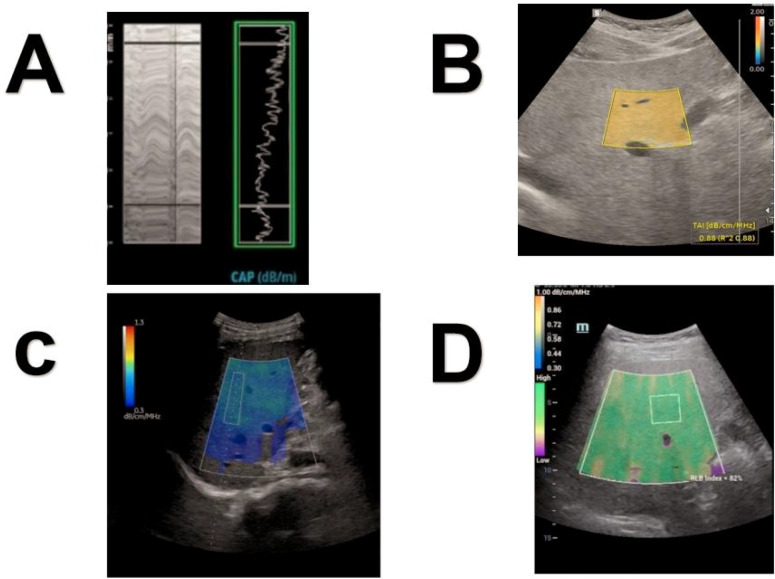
Representative ultrasound images of attenuation-based techniques for hepatic fat quantification. (A) CAP (Controlled Attenuation Parameter); (B) TAI (Tissue Attenuation Imaging); (C) UGAP (Ultrasound-Guided Attenuation Parameter); (D) USAT (Ultrasound Attenuation Analysis). Each modality estimates hepatic fat content by analyzing signal attenuation patterns with or without real-time B-mode image fusion.
